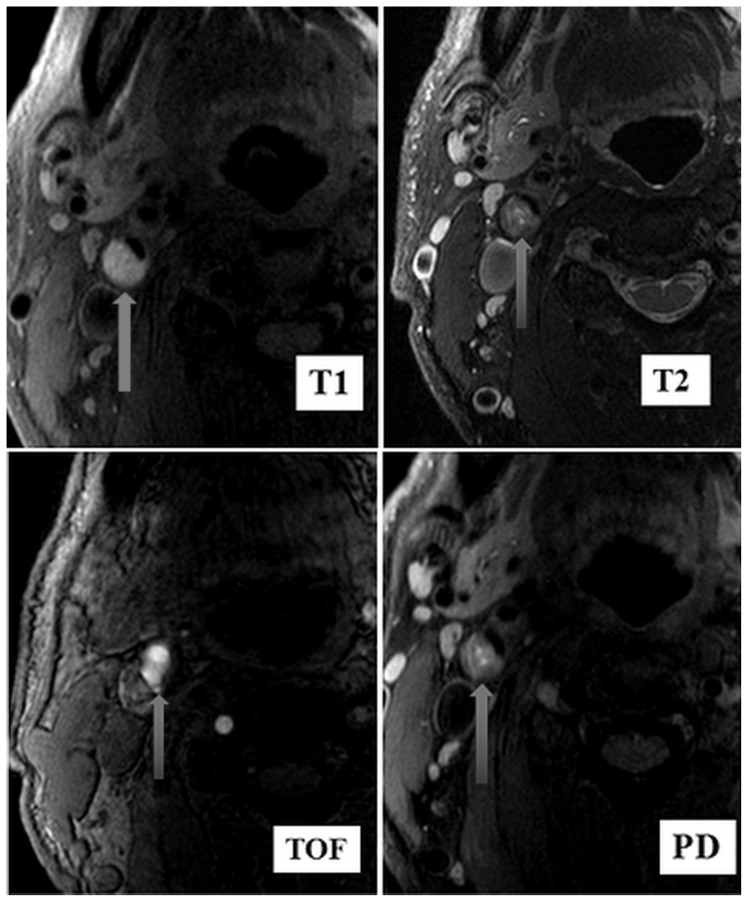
C-HR-MRI revealed vulnerable plaques (type VI: intraplaque hemorrhage). The plaque was presented by the arrow. The C-HR-MRI manifestations of type VI AS (intraplaque hemorrhage) were high-signal T1 and TOF images, as well as high-, iso-, or low-signal T2 and PD images.
